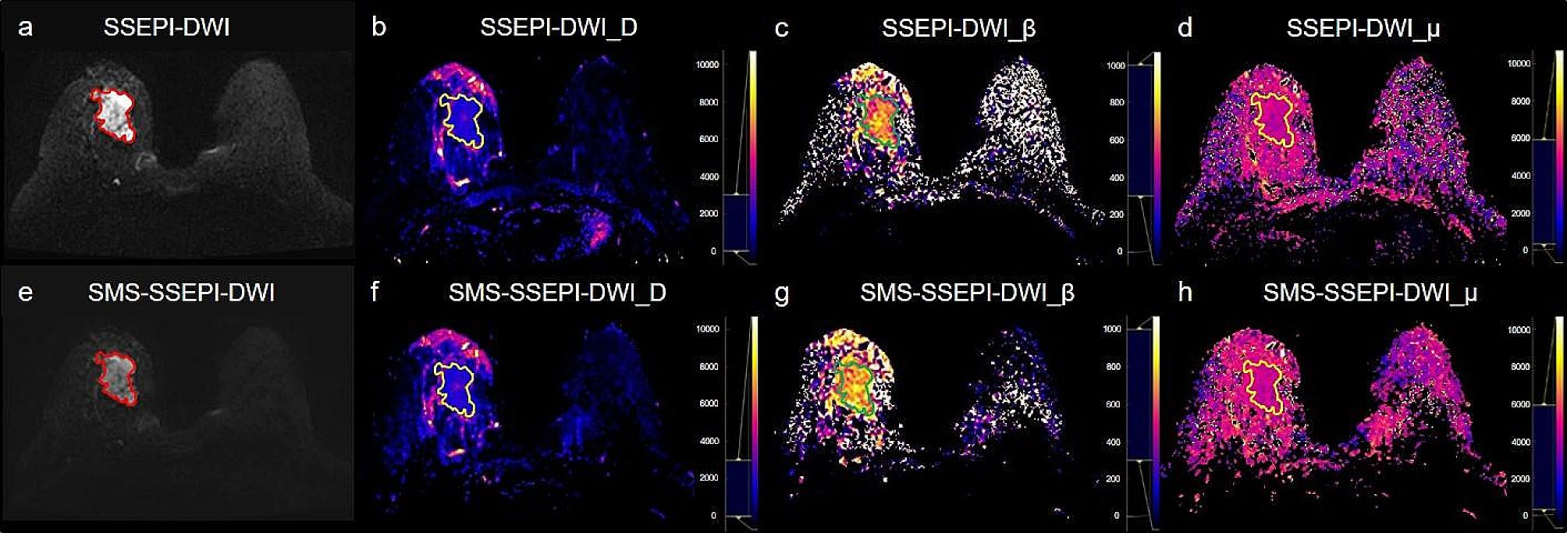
Invasive ductal carcinoma of the right breast in a 54-year-old woman. The solid line area indicates the extent of the lesion. a-d: Conventional SSEPI-DWI image (b = 1000 s/mm²) (a) and quantitative parametric maps (b-d), the SNR and CNR of the lesion area are 64.72 and 8.95 respectively (a), the average values of the parameters within the lesion area are D = 0.85 μm²/ms, β = 0.73, µ = 3.5 μm. e-h: SMS-SSEPI-DWI image (b = 1000 s/mm²) (e) and quantitative parametric maps (f-h), the SNR and CNR of the lesion area are 58.25 and 8.13 respectively (e), the average values of the parameters within the lesion area are D = 0.87 μm²/ms, β = 0.76, µ = 3.6 μm
